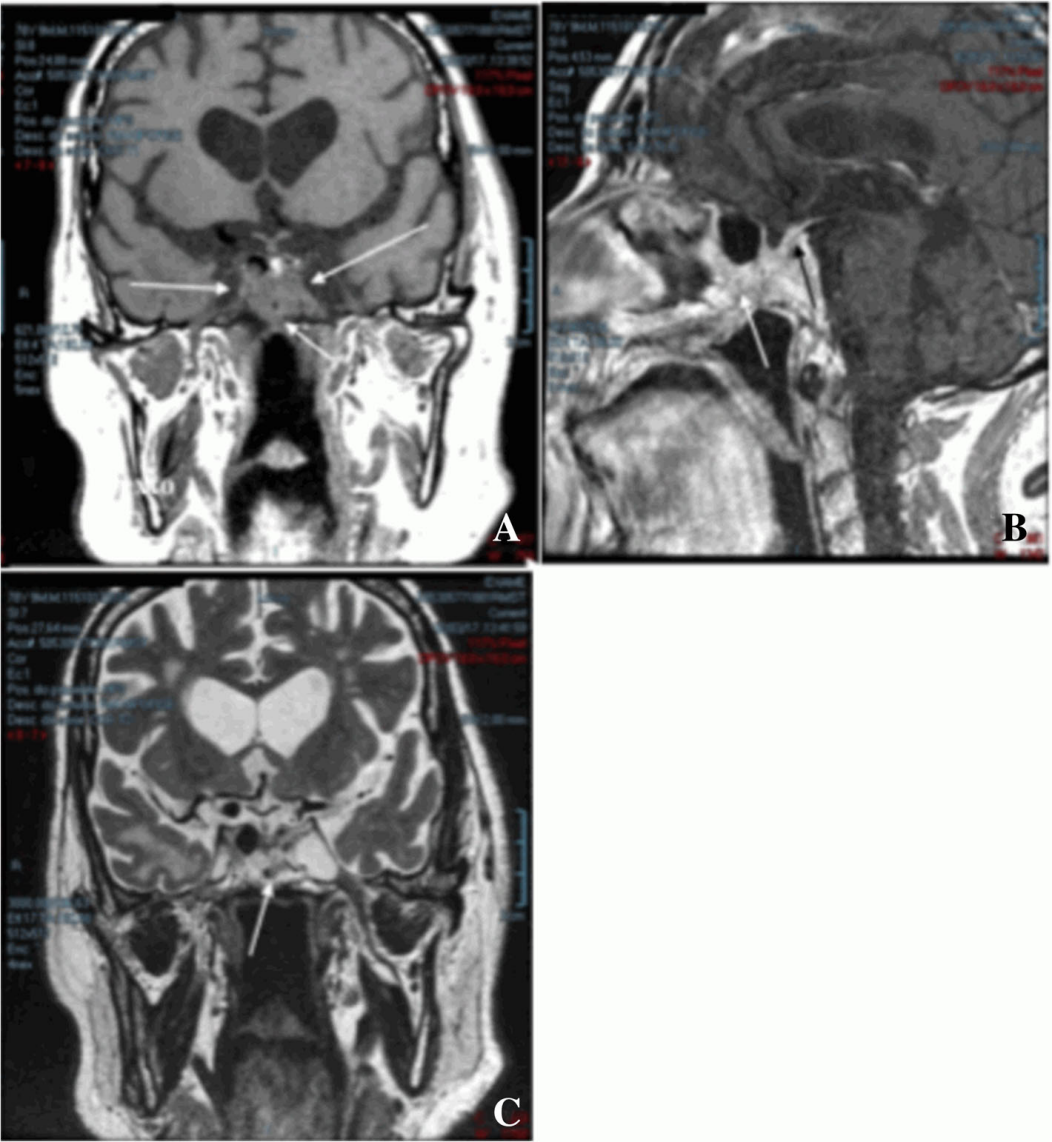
Magnetic resonance imaging of the sella turcica 17 years after starting treatment with cabergoline. a Coronal plane image with heterogeneous contrast uptake confirming the reductions of cavernous and sphenoidal sinus invasions (wh*ite arrows*). b Profile image with heterogeneous contrast uptake demonstrating invasion of the sphenoid sinus (*white arrow*) and with a pituitary stalk capturing contrast (*black arrow*). c Coronal plane image in T2 showing the cystic degeneration of the tumor (*white arrow*)
